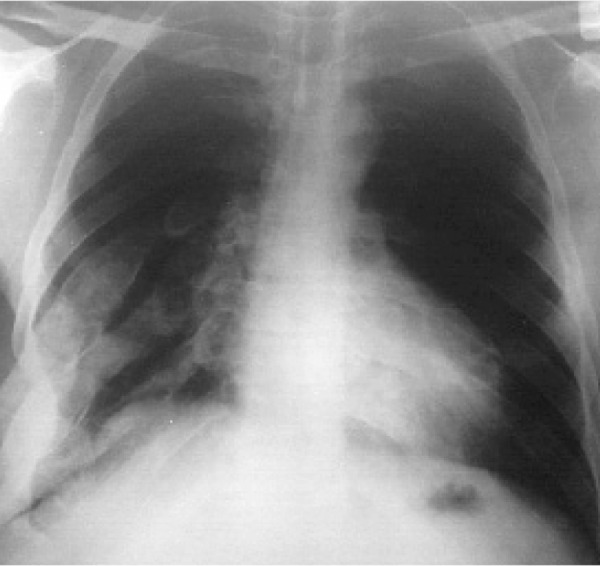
A 20-year-old boy with deformity of the chest.PA chest X-ray
